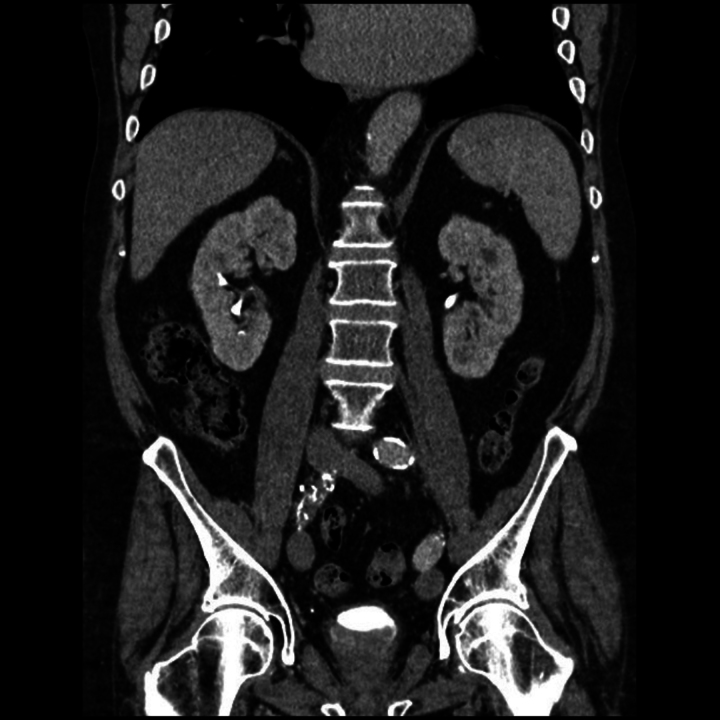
Computed tomography (CT) imaging of the abdomen and pelvis with contrast showing urinary bladder with slightly thickened walls and multiple clots; kidneys showing multiple bilateral small cortical cysts and no hydronephrosis
